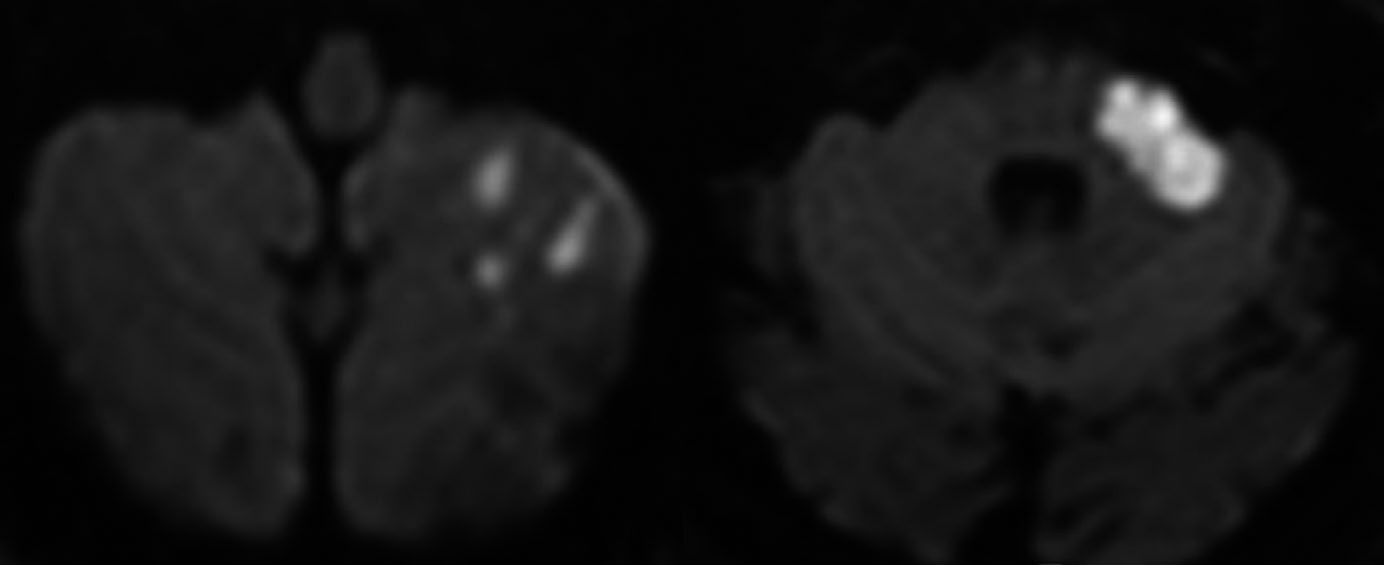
MRI‐DWI showing multiple small areas of restricted diffusion in the inferior left cerebellar hemisphere, and at the level of left middle cerebellar peduncle, also present on fluid attenuated inversion recovery sequence (not shown), suggesting subacute ischemic lesions
